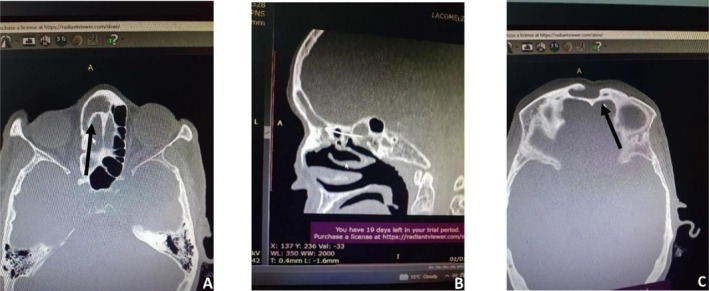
Axial CT scan of the frontal sinus showing an intact posterior table and a hypodense lesion occupying the frontal sinus cavity (A), sagittal CT image showing obstruction of the frontal sinus extending into the frontoethmoidal recess (B), axial CT scan showing a defect in the anterior table of the frontal sinus with a soft tissue density mass occupying the frontal sinus and extending into the frontoethmoidal recess (C).
